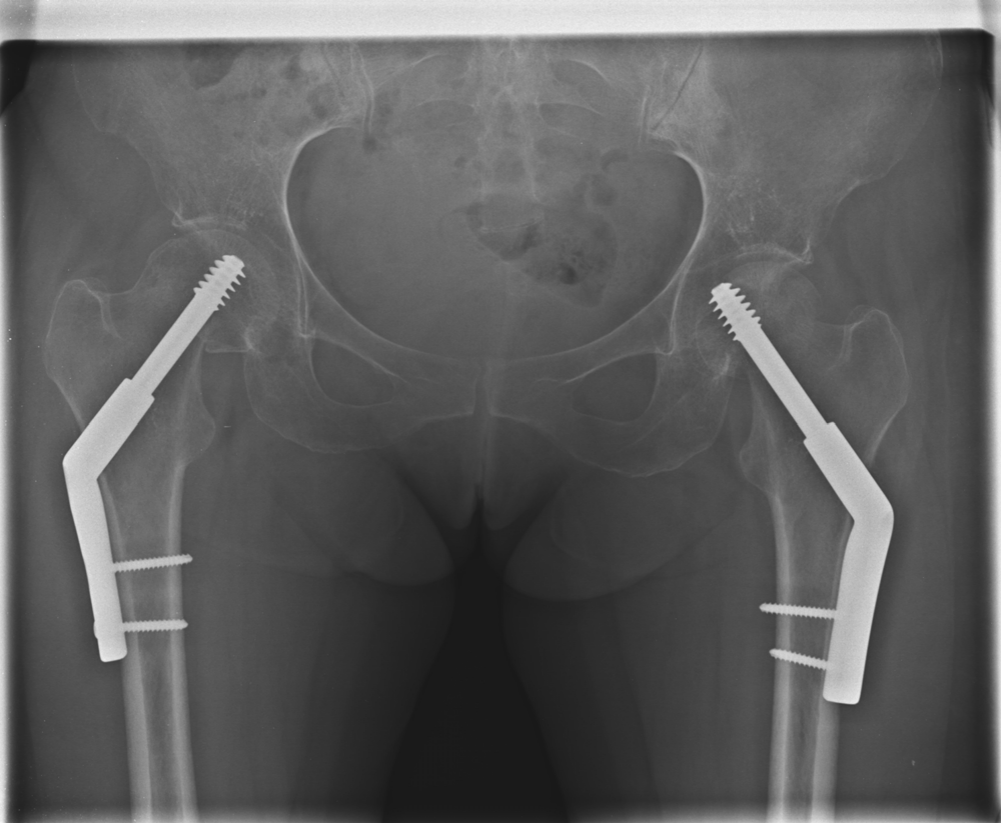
Antero-posterior radiograph of the pelvis post fixation with dynamic hip screws.
